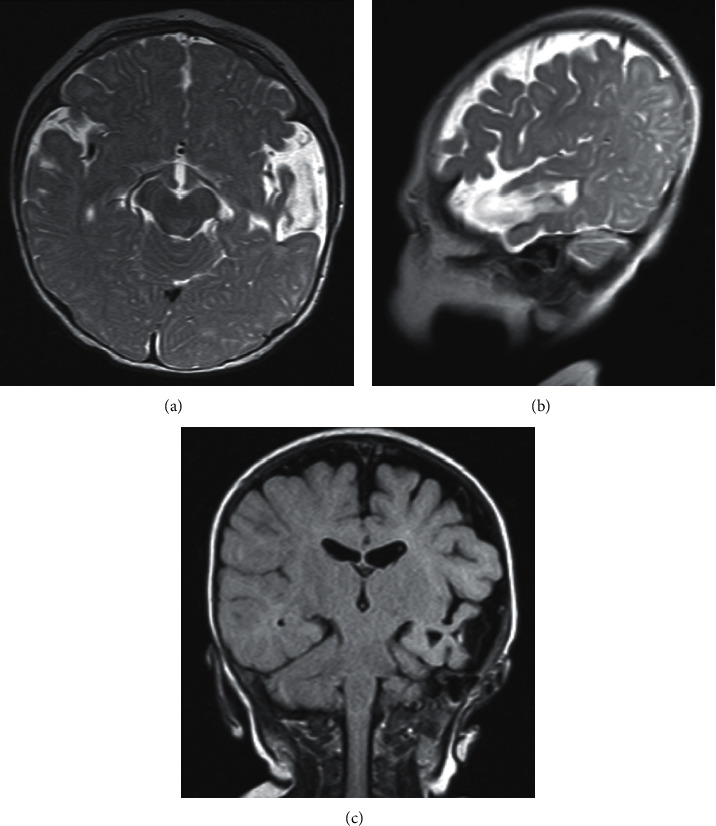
(a)-(c) Brain MRI (T2, T1, and T2 flair images) of the second patient showing left temporal lobe peripheral and periventricular focal encephalomalacia.
